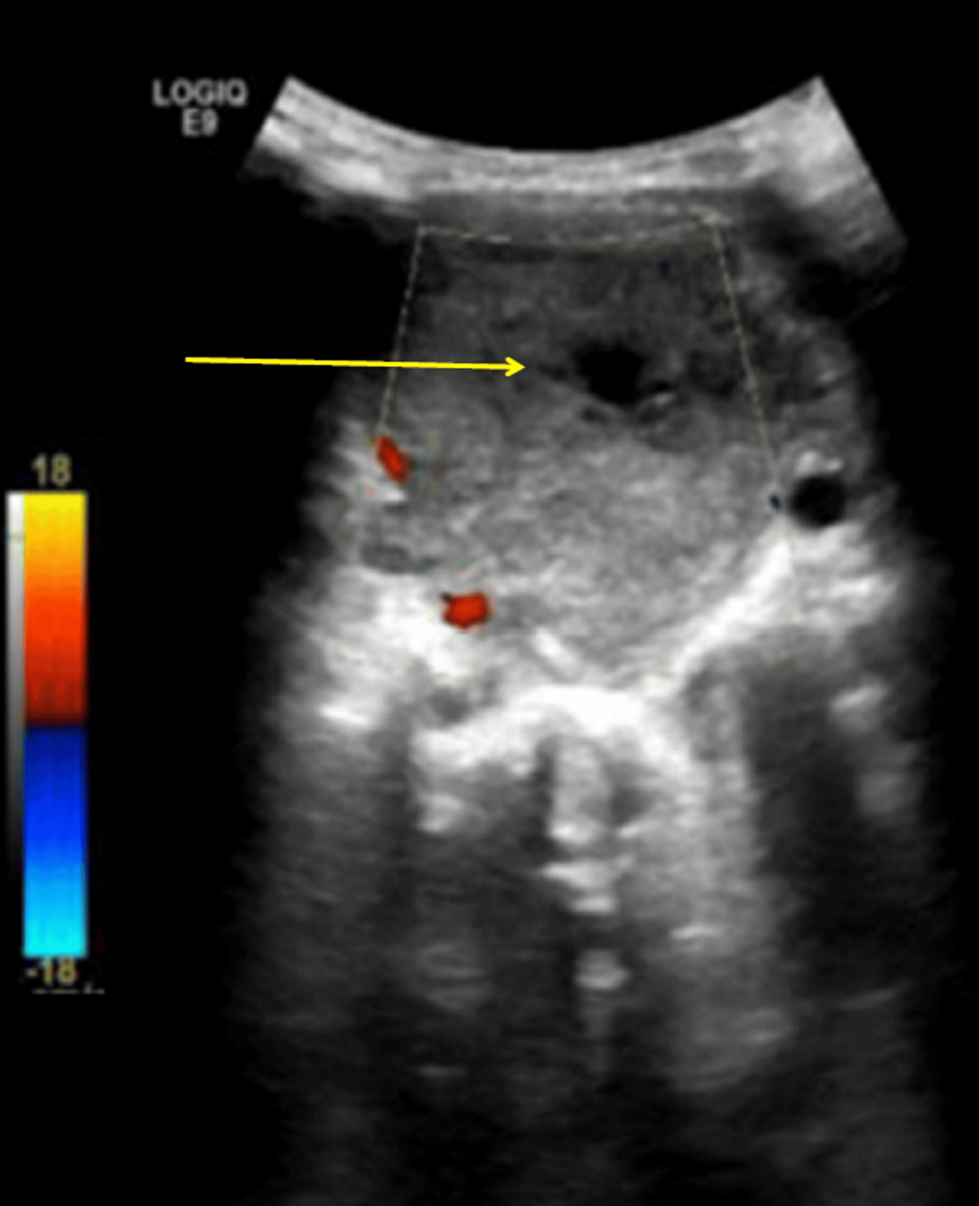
Ultrasound of the neck showing a heterogeneous solid mass with a necrotic center (yellow arrow)
